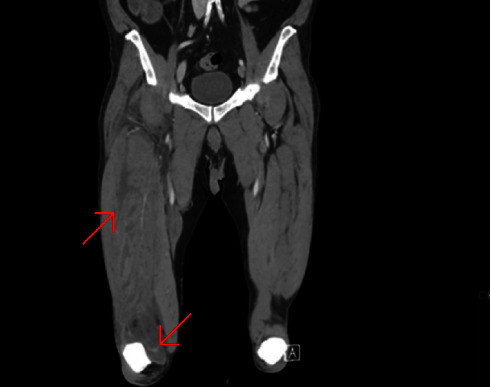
Coronal view of a CT scan, demonstrating an increase in right thigh circumference.
